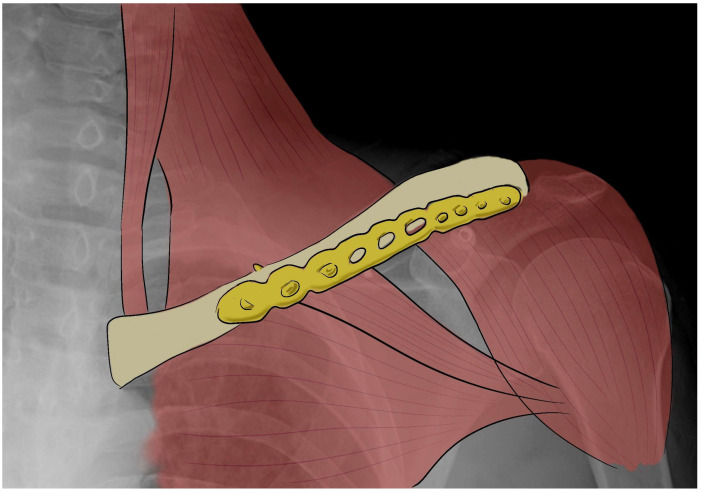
X-ray image with a draw of clavicle fracture fixed by an anteroinferior plate.
